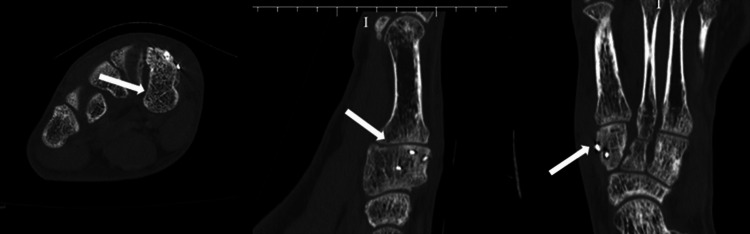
Postoperative CT at 3 months after the operation. Bone union was observed.
